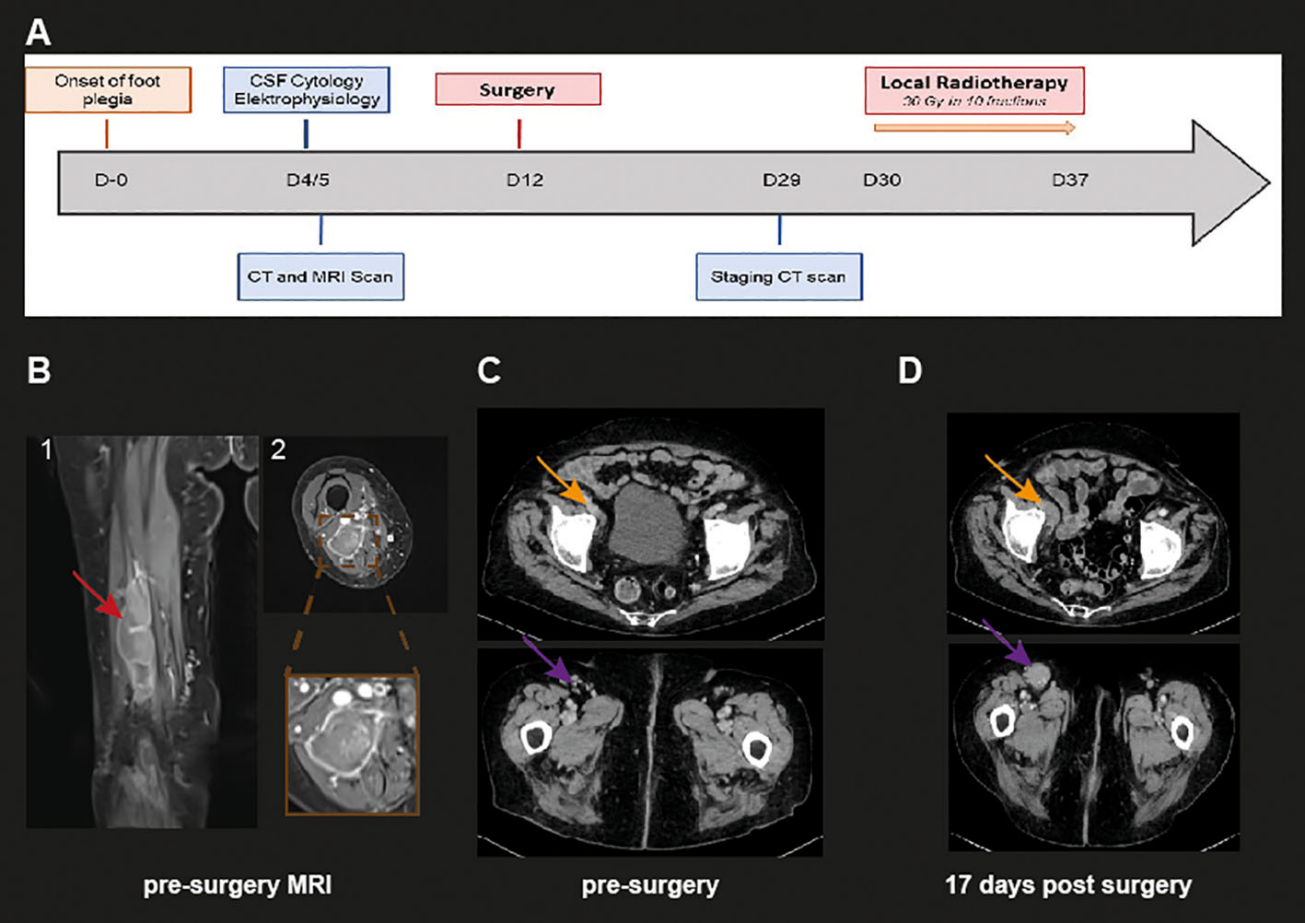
Clinical history and representative radiological images. (A) Clinical course and diagnostic work-up of the described case. Day 0 is defined as the day when paralysis of extension of the right foot was evident for the patient. (B) Representative coronal (1) and axial (2) MRI images of the right tigh, showing an inhomogeneous contrast-enhancing lesion (red arrows). The brown box depicts a close-up image of the axial tumor expansion (C) Representative axial and coronal CT image of the initial CT scan on day 4 revealing no further nodular DLBCL lesions. (D) Representative axial and coronal CT image 17 days post-surgery showing novel malignant lesions in the ventral right femoral compartment (top orange arrow) as well as in the right inguinal region (bottom violet arrow).
